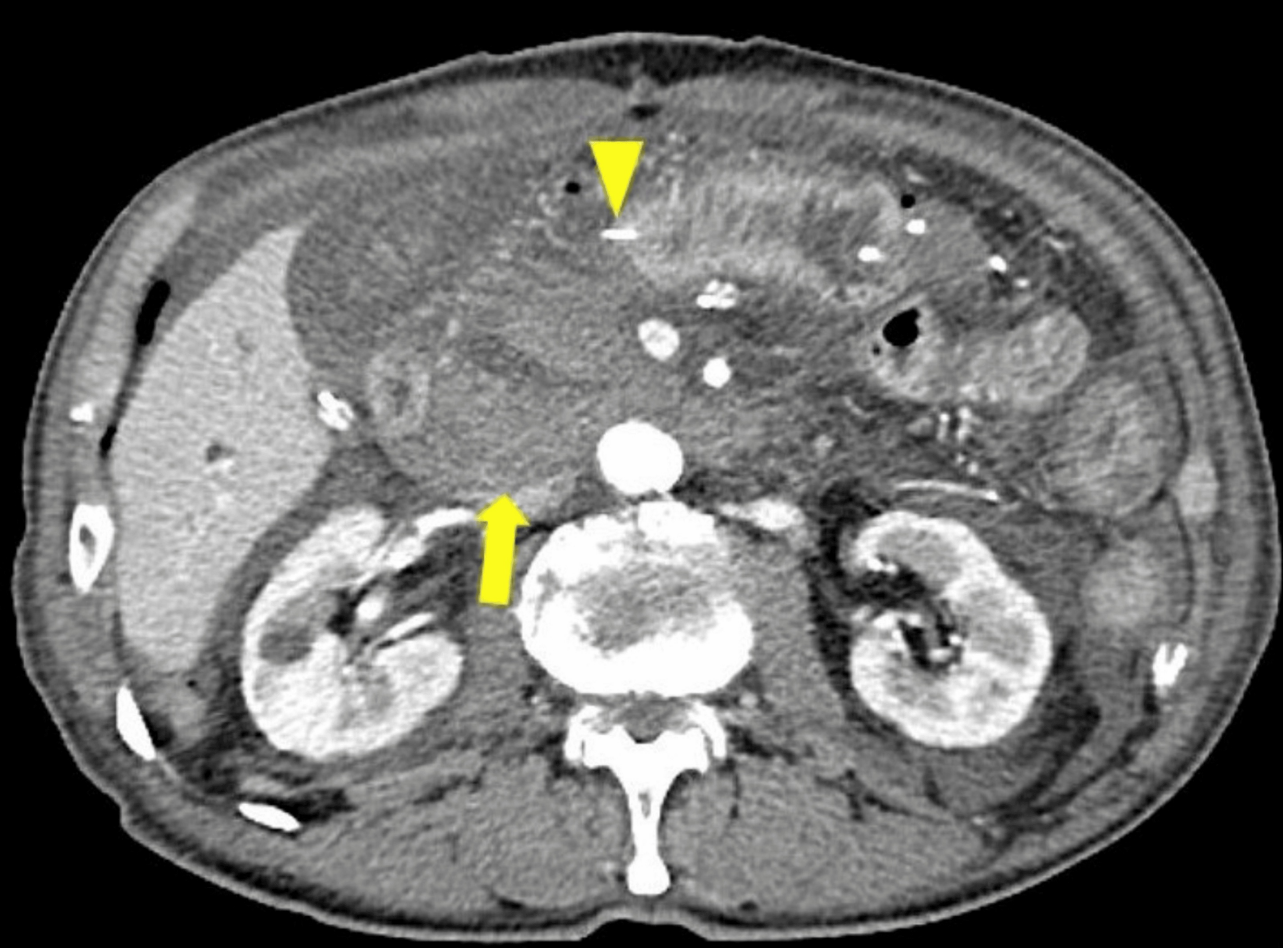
Enhanced abdominal computed tomography of Case 2. Computed tomography showed the pancreatic stent penetrating the jejunum (arrowhead) and a hematoma on the dorsal section of the jejunal loop (arrow).
